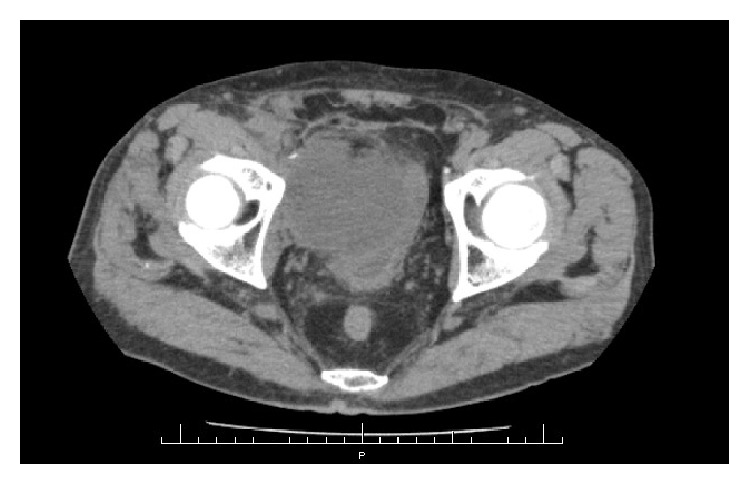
CT scan shows an 80 mm cystic lesion displacing the urinary bladder 6 months after the surgery.
